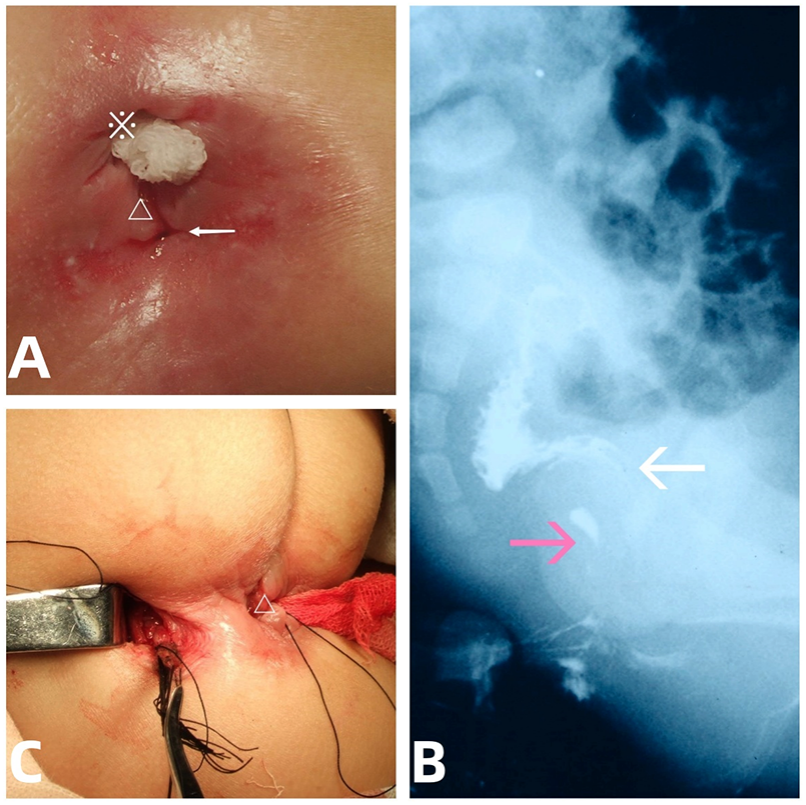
The presentation, fistulogram, and intraoperative view of the ACD. (A) Accssory perineal opening with clear fluid discharge (arrow), perianal erosion, anal fissure (△), and native stenosed anus (※). (B) Lateral abdominal radiograph showing a contrast medium outlining a tubular duplication (pink arrows) without communication to the native anorectum (white arrows). (C) Removal of the tubular mucosa (arrow) via the perineal (△) combined with coccygeal transverse approach.
